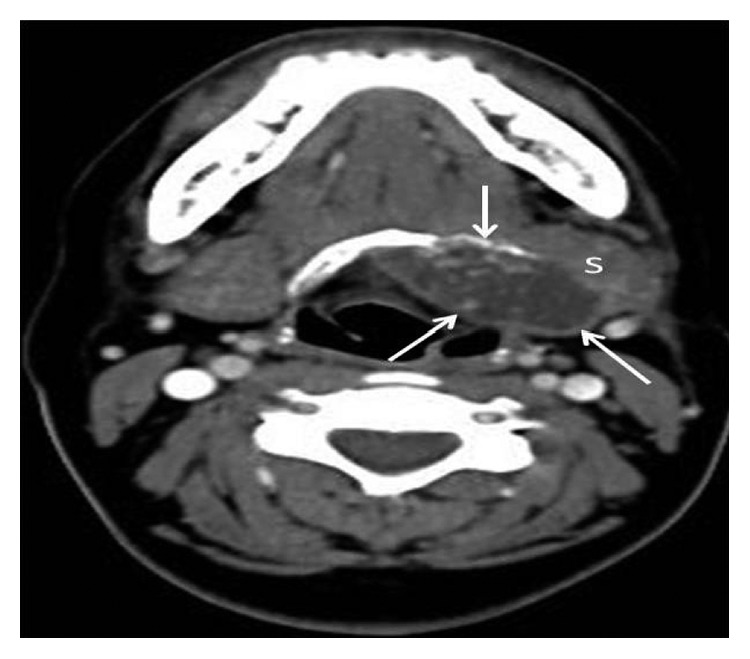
Contrast-enhanced axial (CT) image: expansile mass originating from the left side of the hyoid bone with cystic-necrotic components and calcific foci (arrows). The mass produces compressive effect on the left submandibular gland (S) and vallecula.
